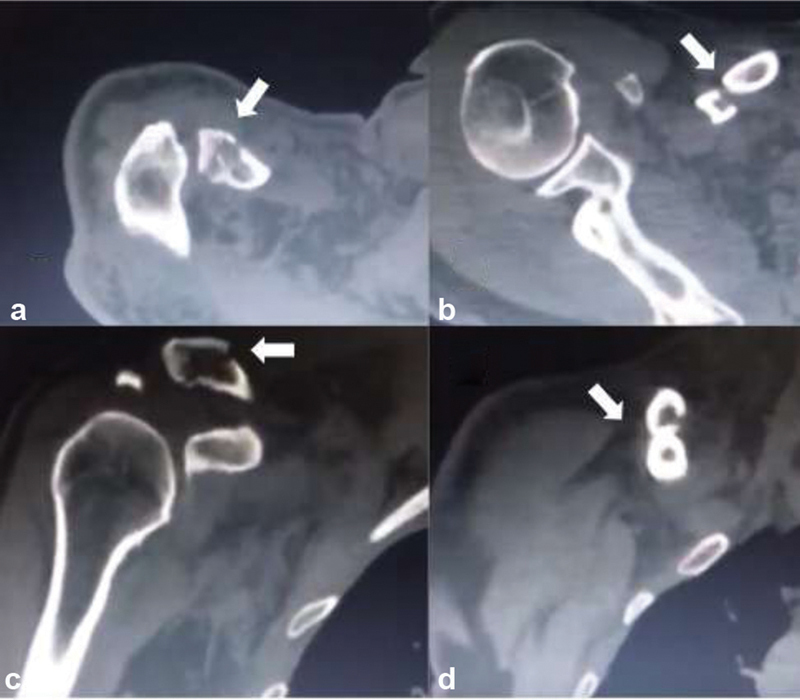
Computed tomography scan of Robinson type 2B2 segmental fracture (a) and (c) Axial and coronal views, lateral fracture (arrow). (b) and (d) Axial and coronal views, middle fracture (arrow).
